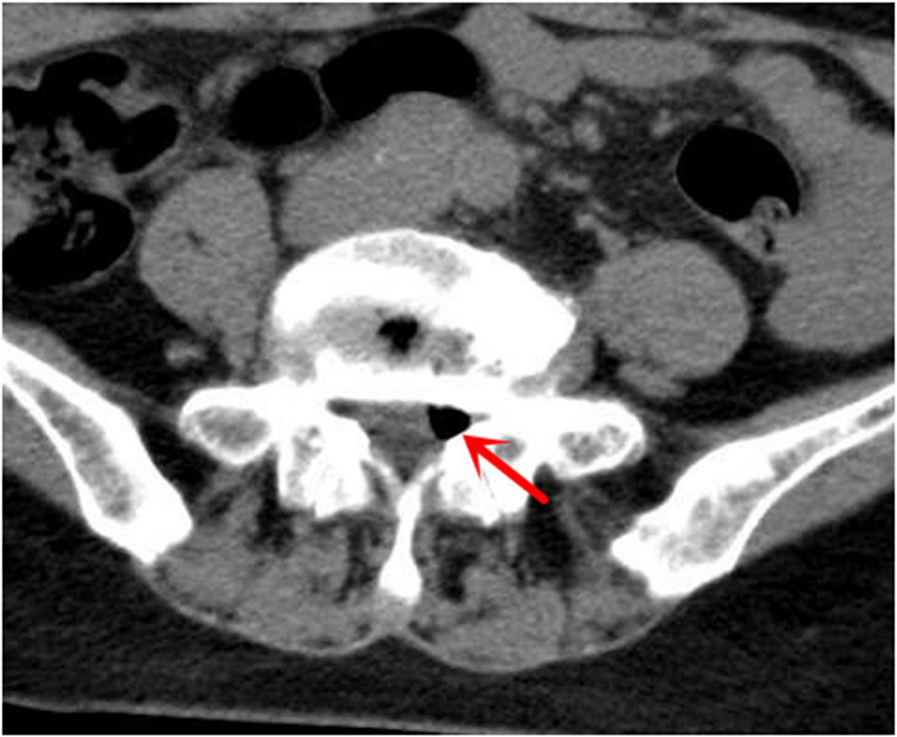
Computed tomography scan reveals low-density mass (red arrow)
